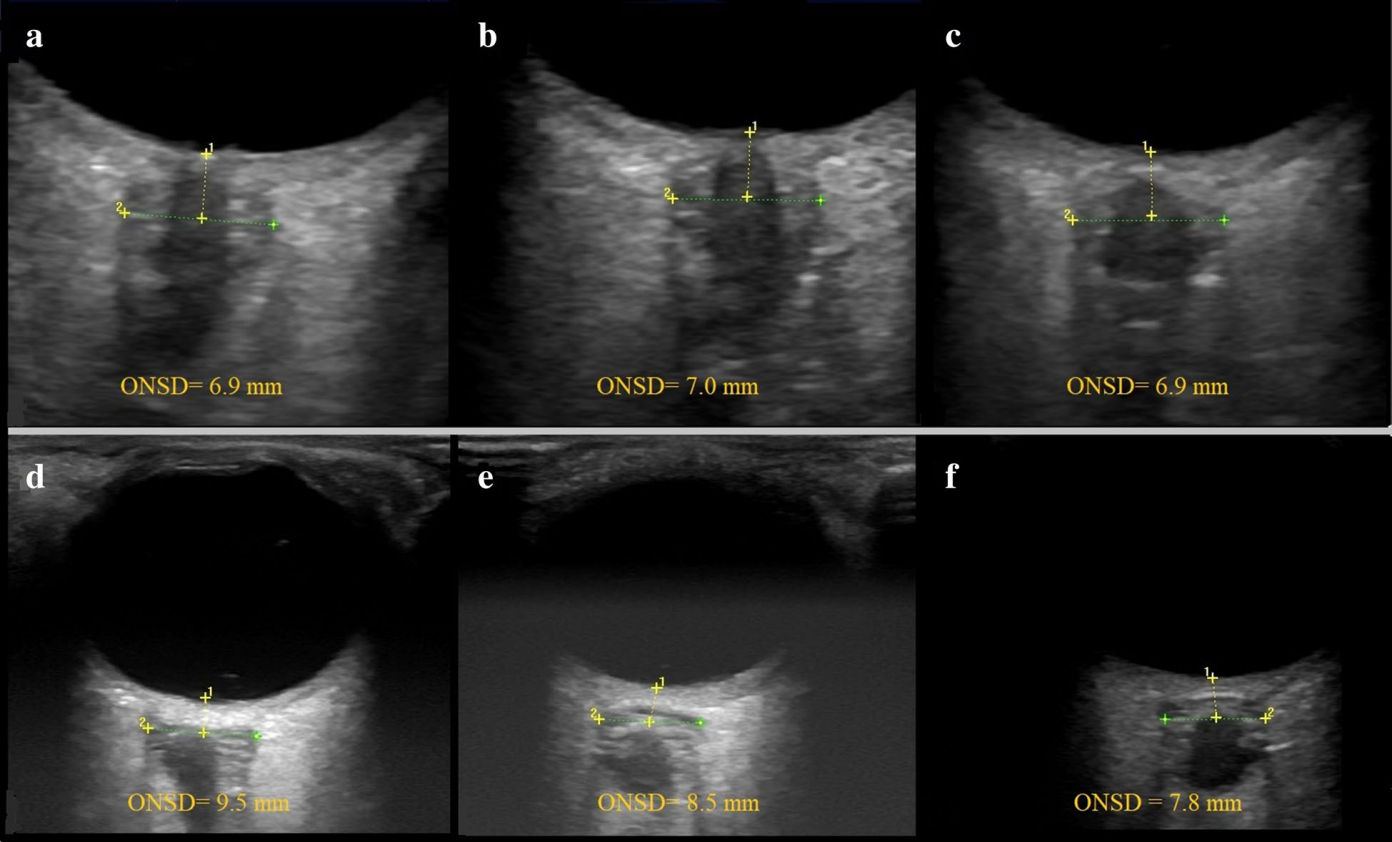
An example of different ONSD trends during the acute stage of ICH. ONSD ultrasound in a patient with ICH performed at admission (a), on the second (b), and the third day (c), representing the stable ONSD trend and example of unstable ONSD trend in another patient (d–f) (*ONSD* optic nerve sheath diameter)
